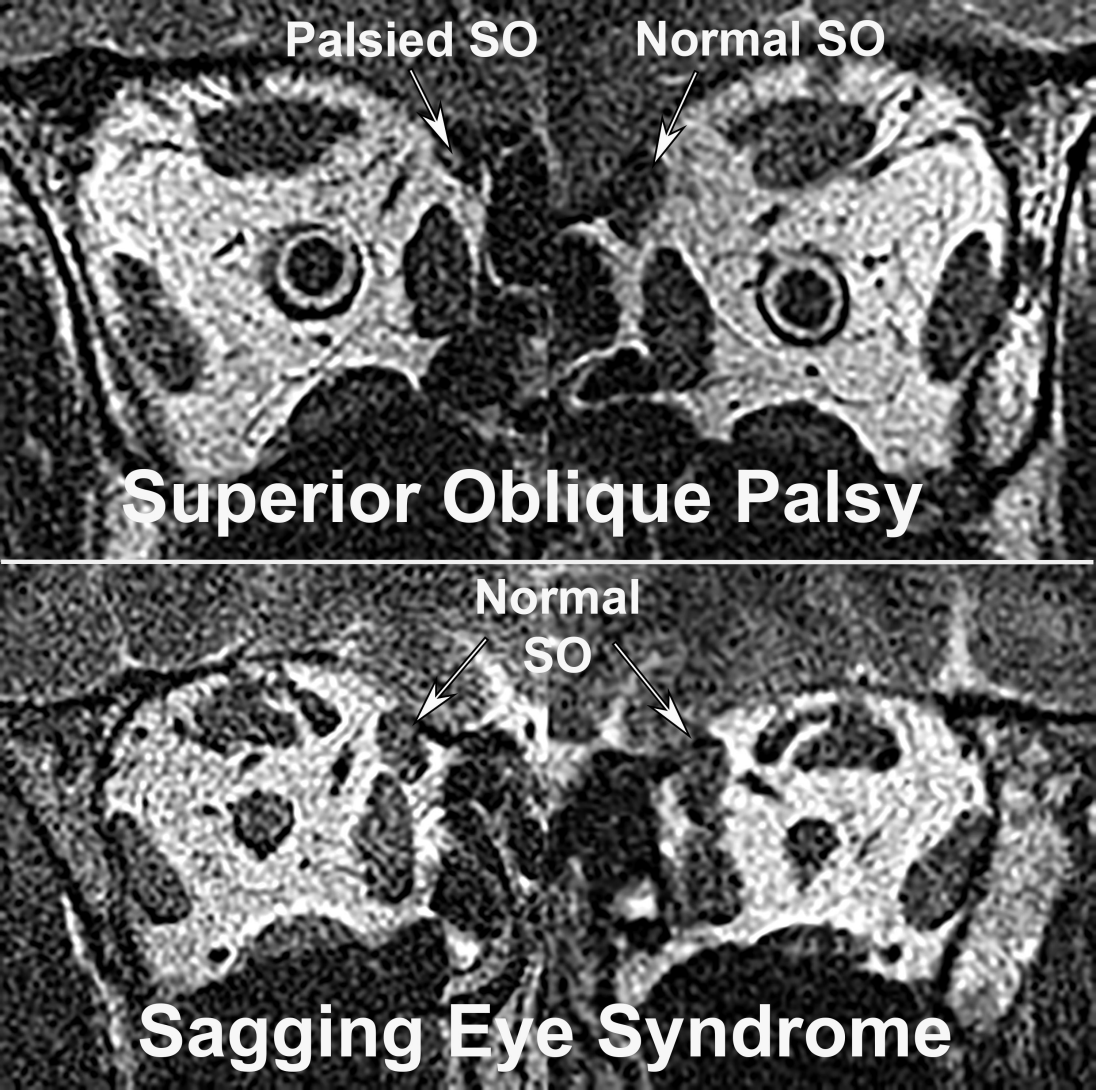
Quasi-coronal MRI in mid-orbital MRI planes in two subjects with right hypertropia due to (A) right SOP, demonstrating reduced right SO cross-section in central gaze, and (B) SES with normal SO cross-sections. See Figure 2 for anterior images. LPS, levator palpebrae superioris muscle; MR, medial rectus muscle.
